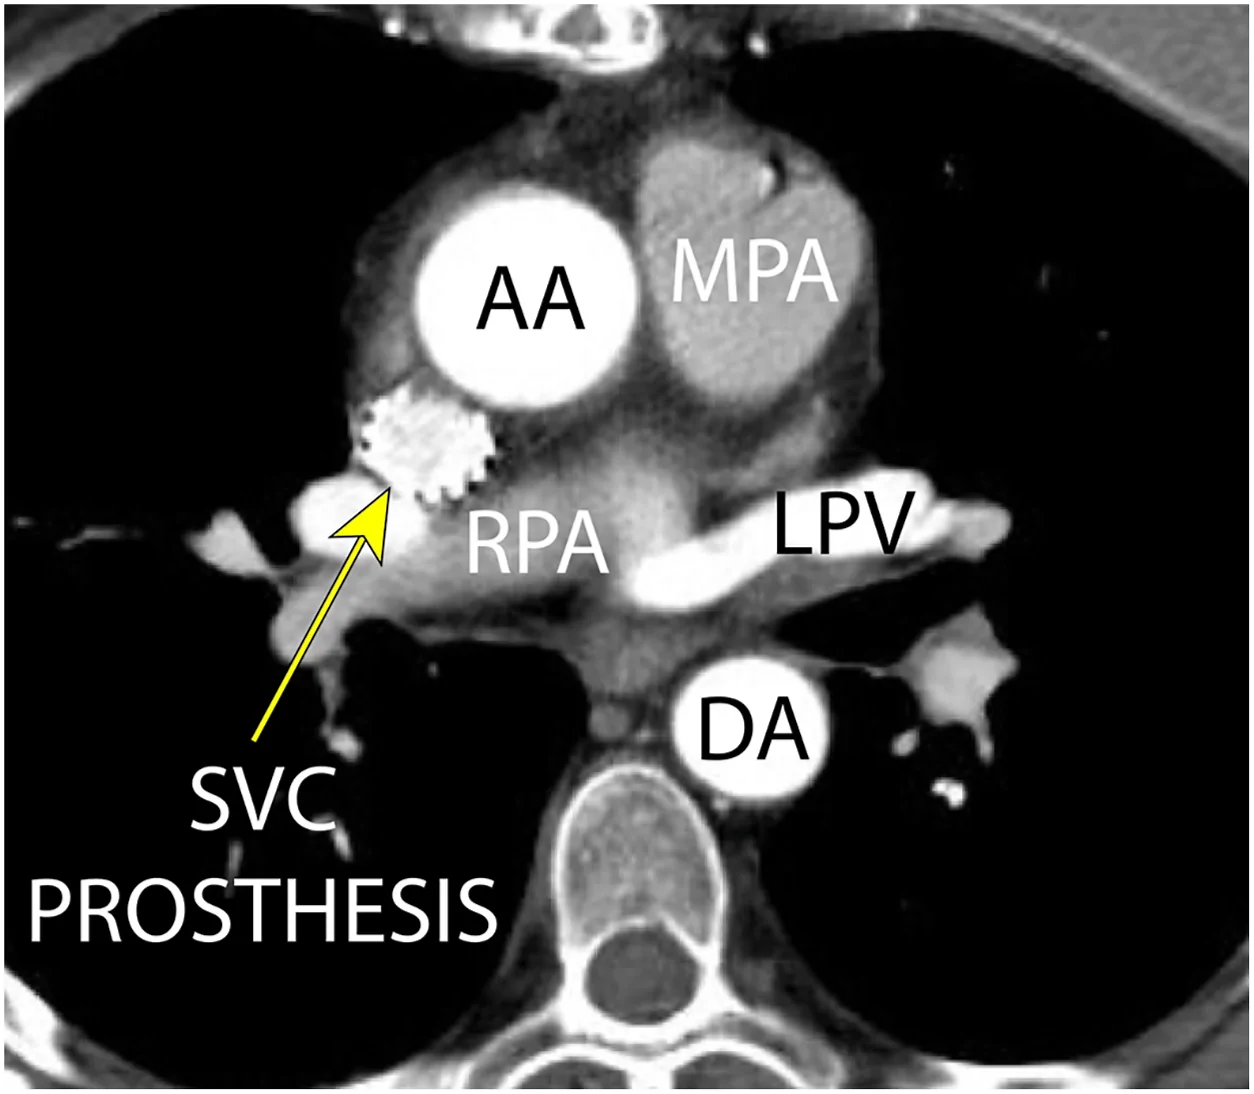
Follow-up imaging. Contrast-enhanced axial computed tomography scan 6 months after surgery showing the prosthesis of the superior vena cava (SVC) (yellow arrow) and the 2 Rosai-Dorfman disease lesions that are morphologically stable. (AA, ascending aorta; DA, descending aorta; LPV, left pulmonary vein; MPA, main pulmonary artery; RPA, right pulmonary artery.)
